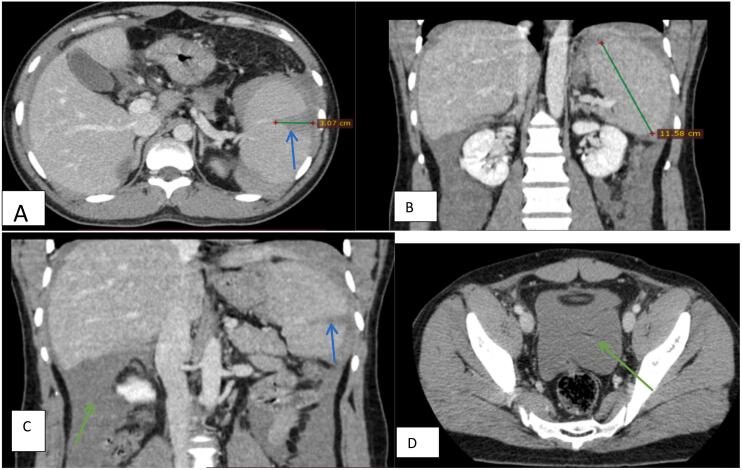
Contrast-enhanced CT of the abdomen. a) Axial, and coronal CT scan at portal venous phase shows: linear hypo dense extending from the inferior splenic capsule to the splenic cortex measuring 3 cm, (blue arrows in a and c images) *peri* splenic and splenic capsule hematoma is identified (a and c). b) Mild splenomegaly measuring 11.5 cm in CC measurement. c) hemoperitoneum around the liver and pelvic seen (orange arrows in C and D images). (For interpretation of the references to color in this figure legend, the reader is referred to the web version of this article.)
